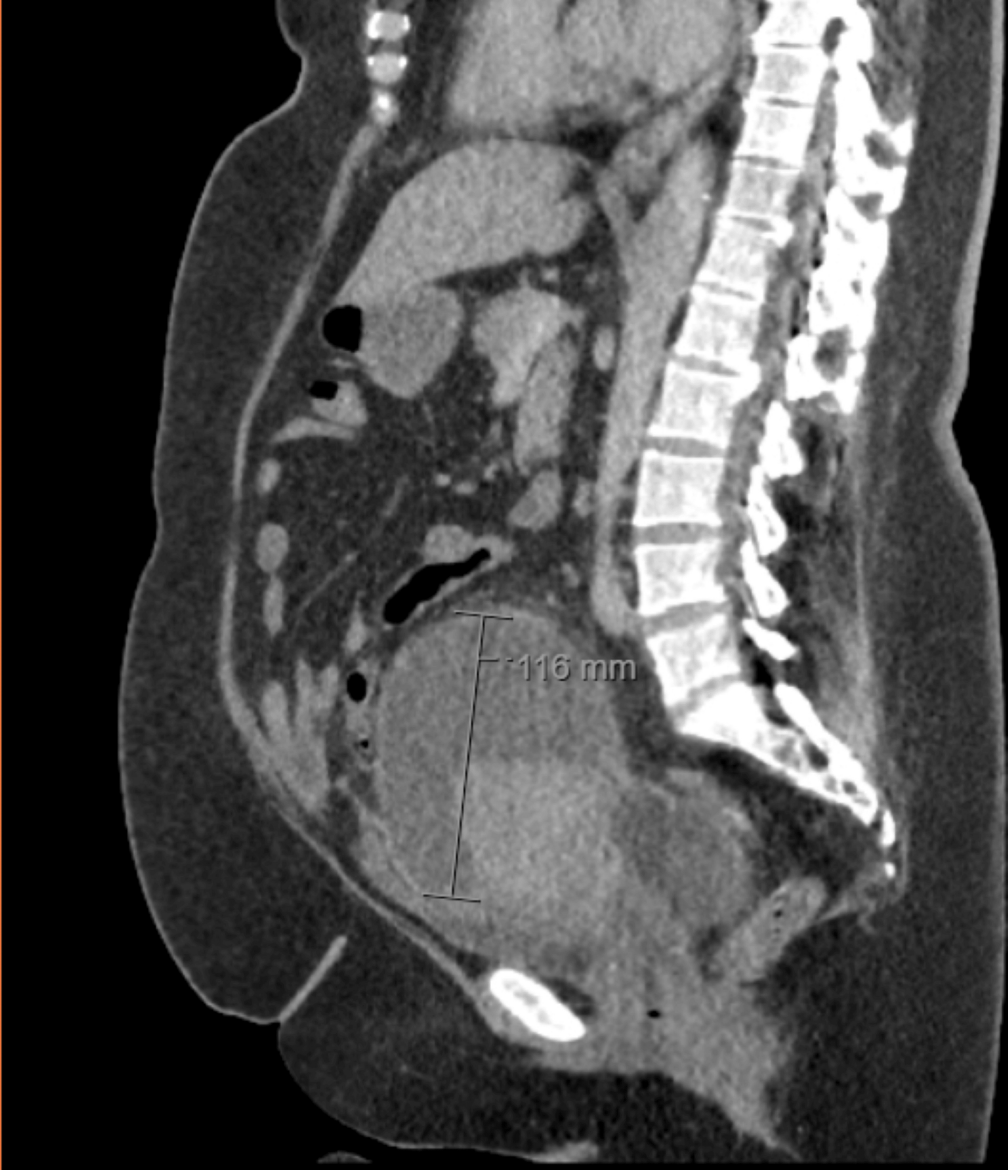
CT scan showing a 116 mm fluid collection (tubo-ovarian abscess)
